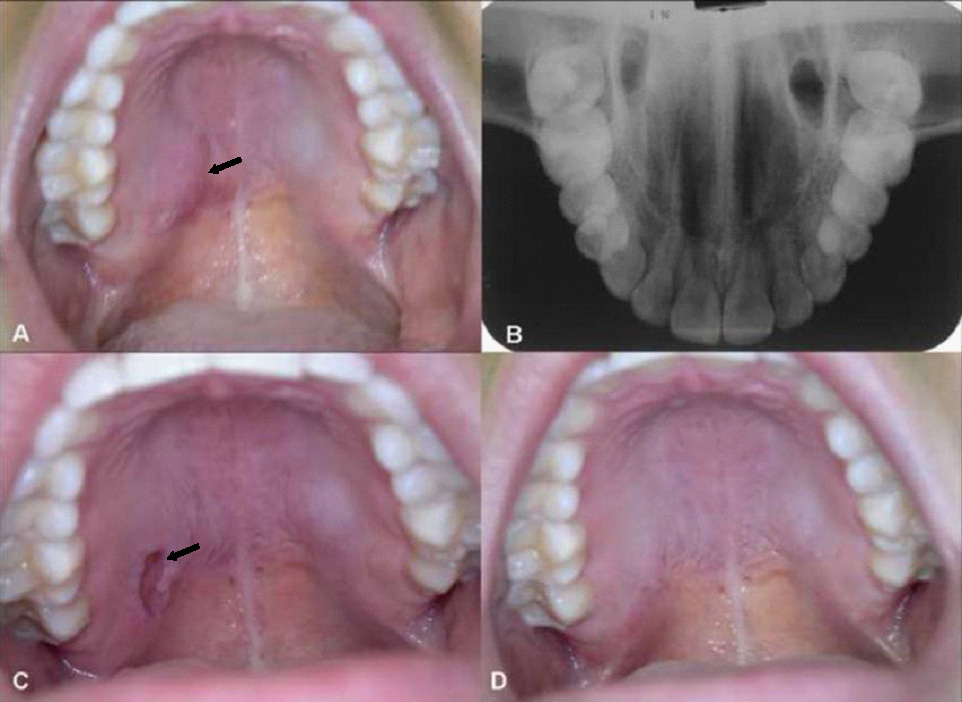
Clinical features. A: Submucosal nodule on the right side of the hard palate in the absence of mucosal alterations (continuous arrow). B: Occlusal radiograph showing no abnormalities. C: Ulceration in the biopsy area after 14 days (continuous arrow). D: Healed area after 30 days.
